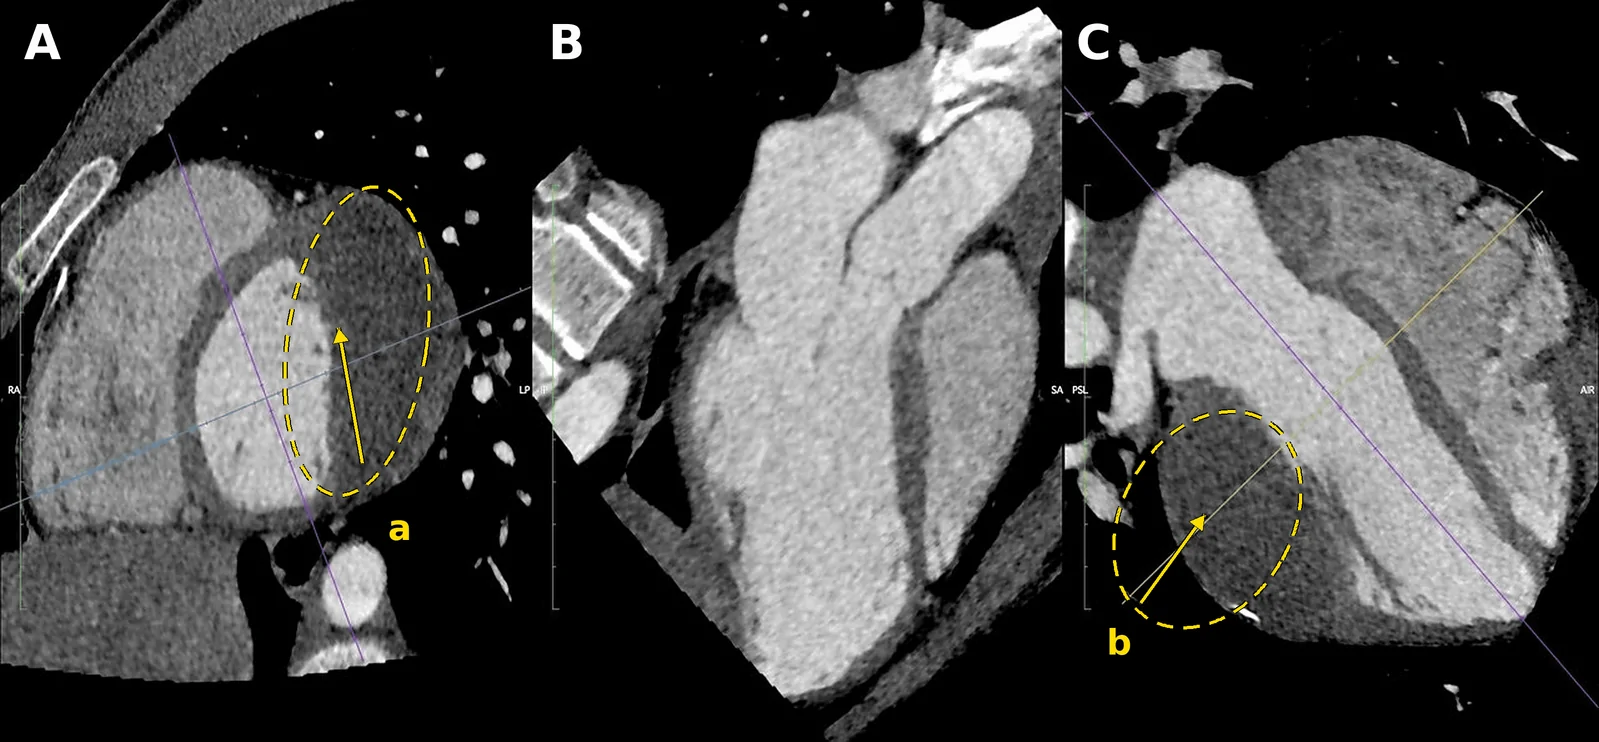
Computed tomography definition of tumour extent Short-axis (A) and orthogonal long-axis multiplanar reformatted images (B and C) demonstrate a 60 × 32 × 53 mm intramural mass arising from the anterior, anterolateral, and inferolateral left ventricular walls. The mass has attenuation similar to adjacent myocardium with focal internal calcification, compresses the left ventricular cavity without causing inflow or outflow tract obstruction, and spares the interventricular septum. A thin residual endocardial myocardial rim overlies the tumour. Yellow dashed outlines and arrows (a, b) delineate the intramural fibroma. CT, computed tomography.
